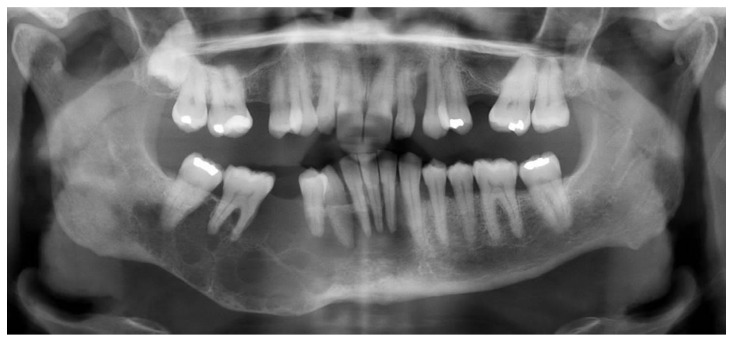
Panoramic X-ray film revealing a 10 cm expansile multilocular radiolucency with poorly demarcated borders in the body of the right mandible.
